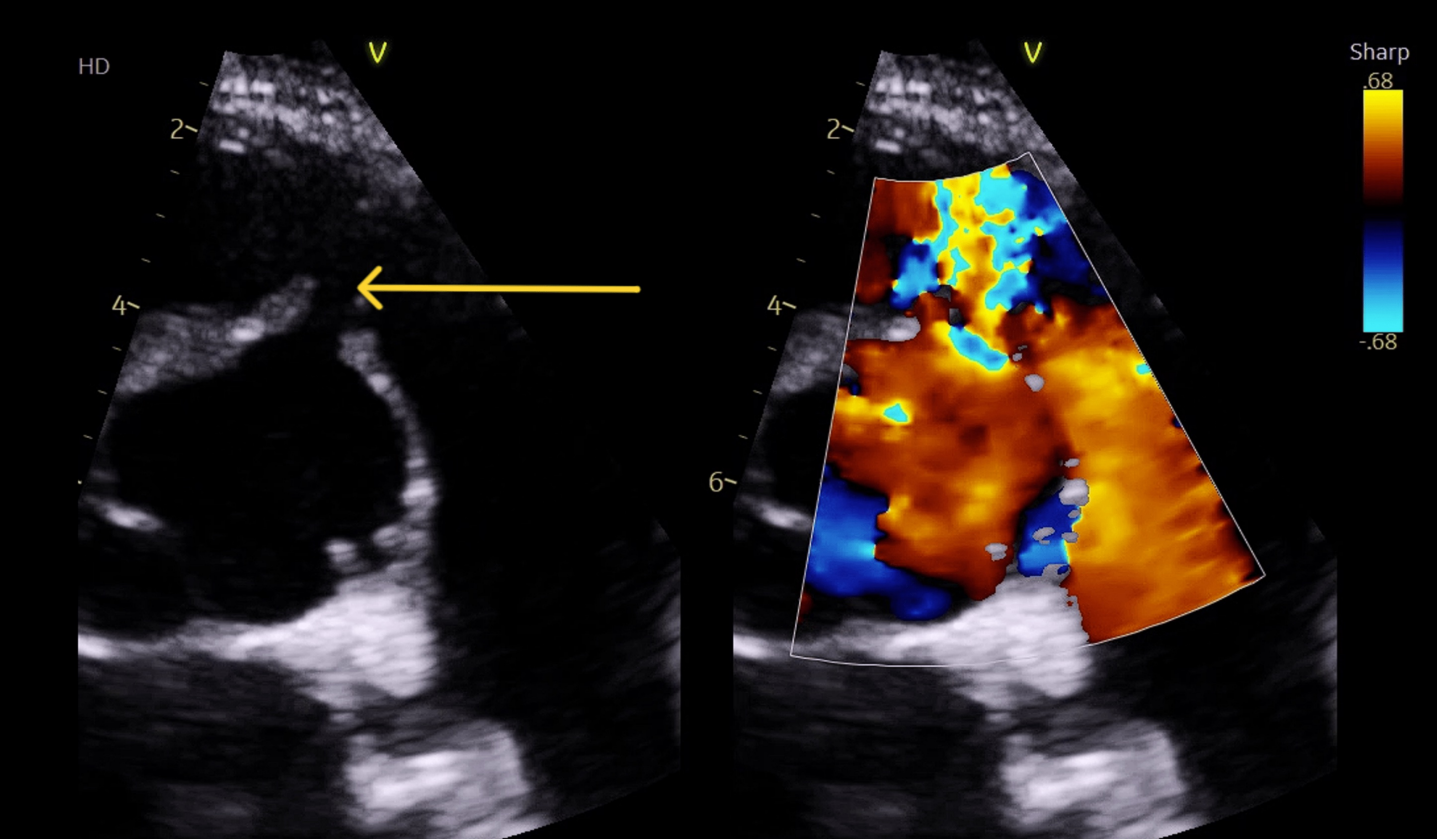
Transthoracic echocardiography demonstrating abnormal turbulent left-to-right color flow at the level of the aortic root.
